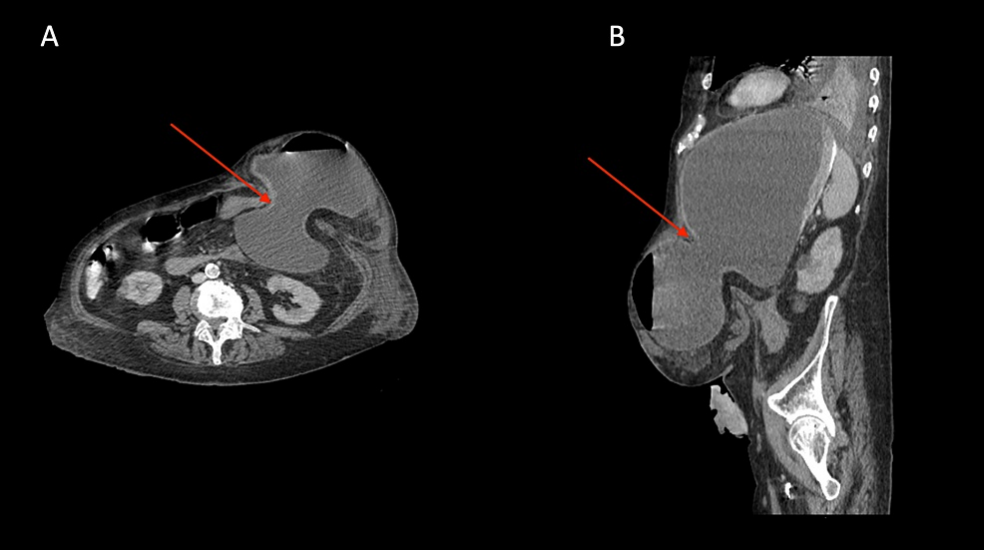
Computed tomography of abdomen and pelvis revealing efferent loop at the neck of the hernia A - Axial view of pylorus emerging as an efferent loop at the hernial neck B - Sagittal view of pylorus emerging as an efferent loop at the hernial neck
